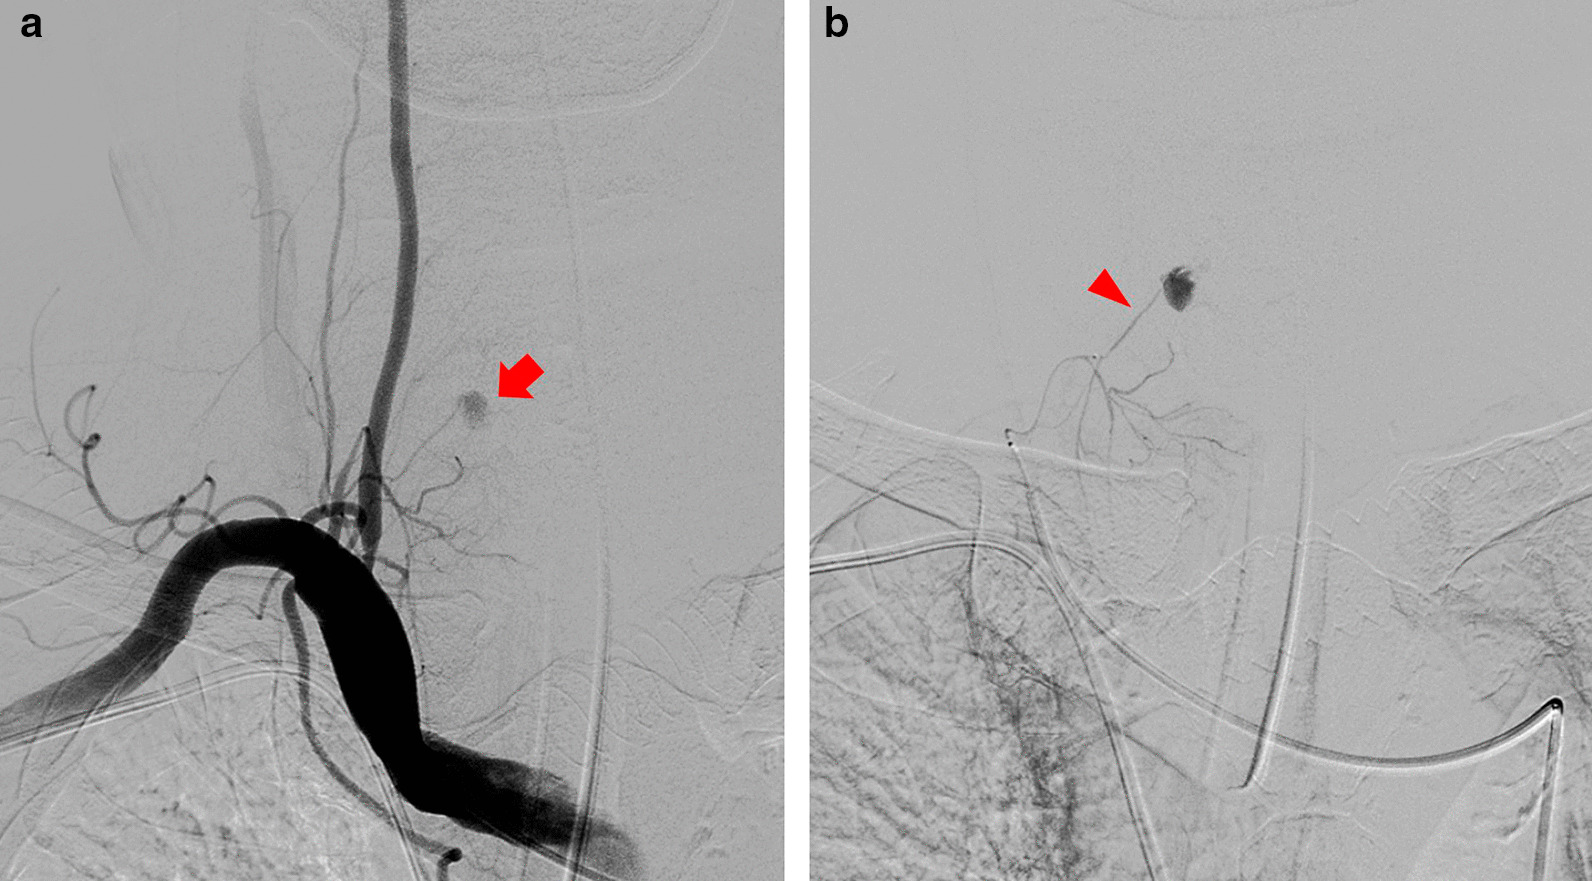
a Right subclavian angiography shows active bleeding (red arrow) in the neck, corresponding to the CT image. b Right thyrocervical trunk is selected and the culprit branch is identified as the right inferior thyroid artery (red arrow head)
